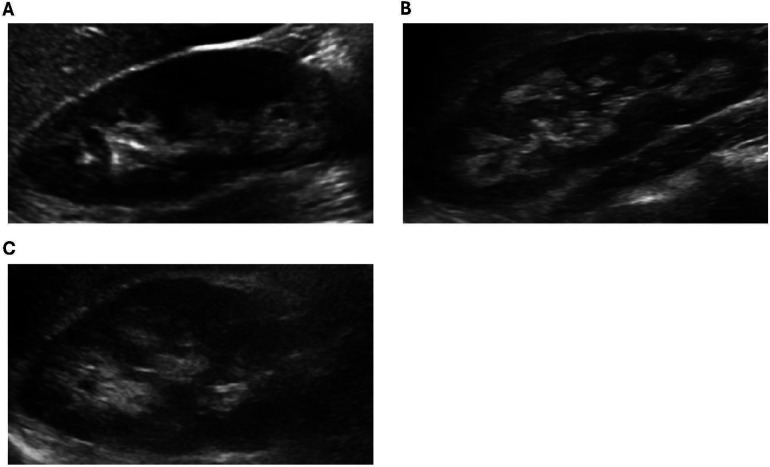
Representative ultrasound images from XLH study patients with NC. (A) Patient 1: increased echogenicity surrounding the medullary pyramids (grade 1). (B) Patient 2: increased echogenicity around and starting to incorporate inside the pyramids (grade 2). (C) Patient 3: increased echogenicity around and starting to incorporate inside the pyramids (grade 2).
